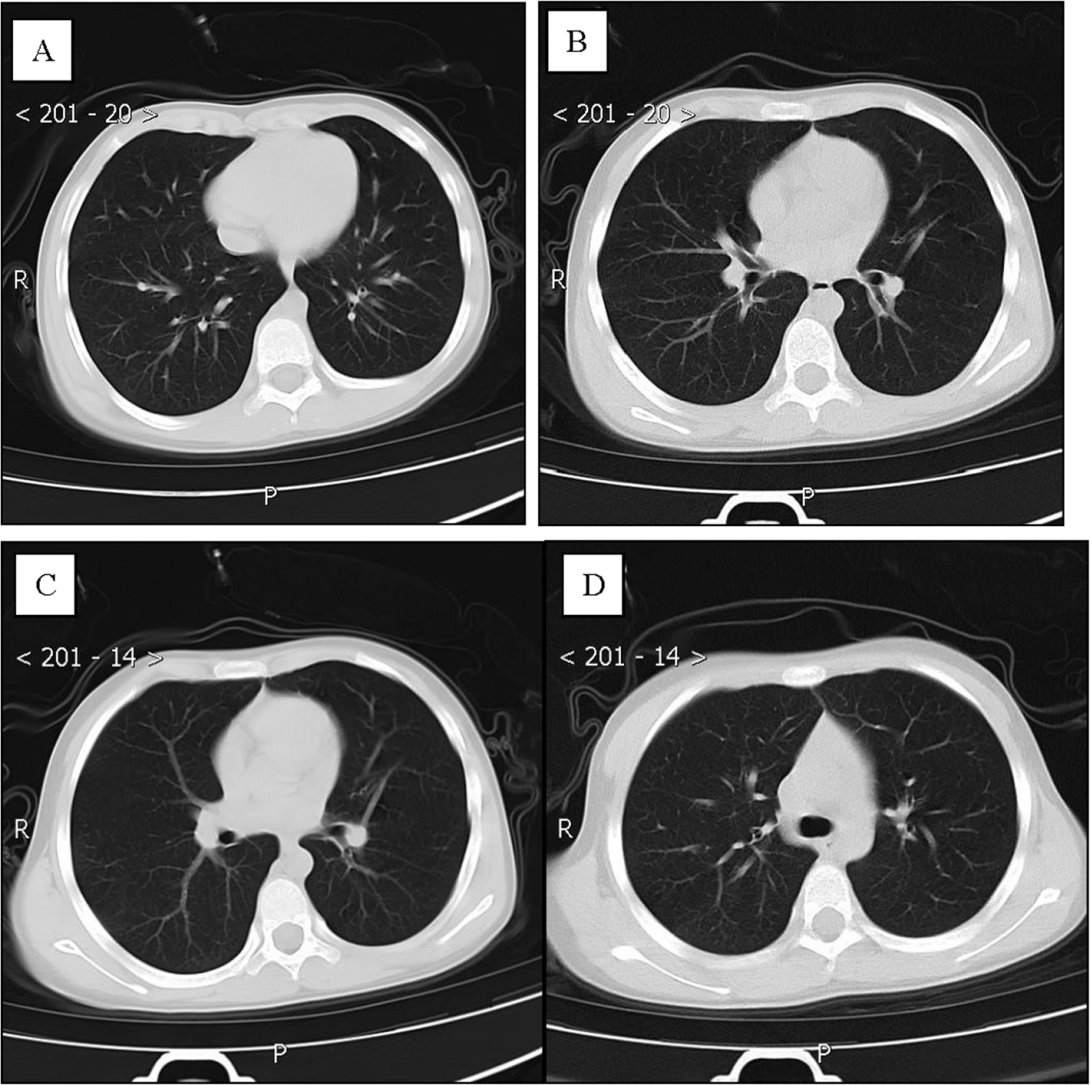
Chest CT images of A 10-year-old boy with COVID-19 infection. a, c Transverse chest CT images showed no abnormalities in both lungs on admission. b, d Transverse chest CT images showed no abnormalities in both lungs on discharge
